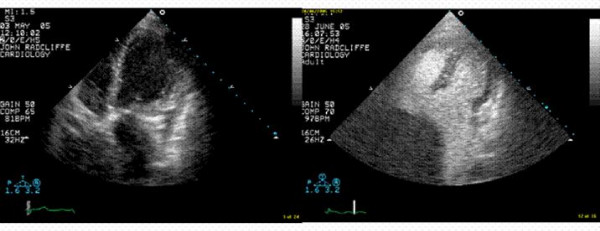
Transthoracic 4 chamber echocardiogram demonstrating noisy native recording with poor visualization of the right atrium. B Transthoracic 4 chamber contrast echocardiogram demonstrating large mass superior to right atrium.
